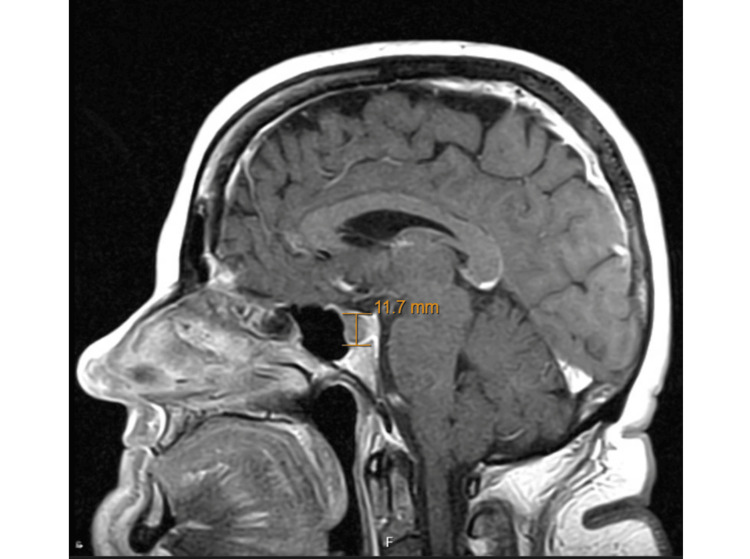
Sagittal MRI obtained four years before the initial endocrine clinic visit, demonstrating an 11.7 mm hypoenhancing lesion within the adenohypophysis with slight suprasellar extension and no evidence of mass effect.
